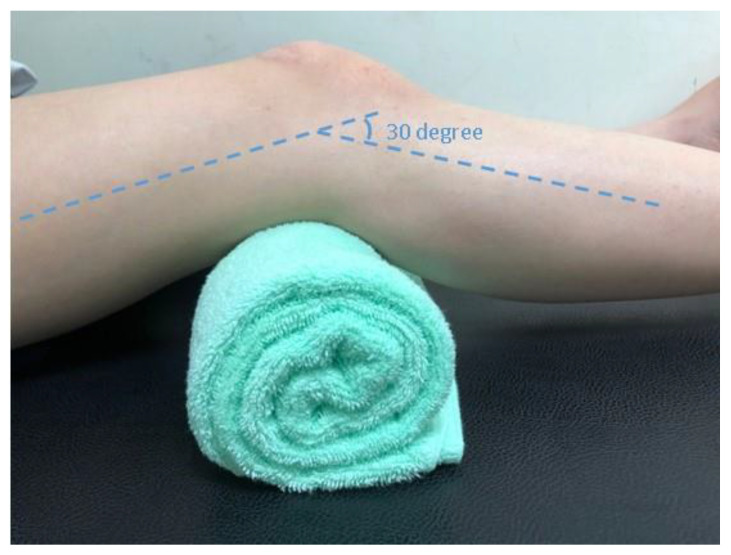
Knee positioned at 30 degrees of flexion for ultrasound evaluation.
